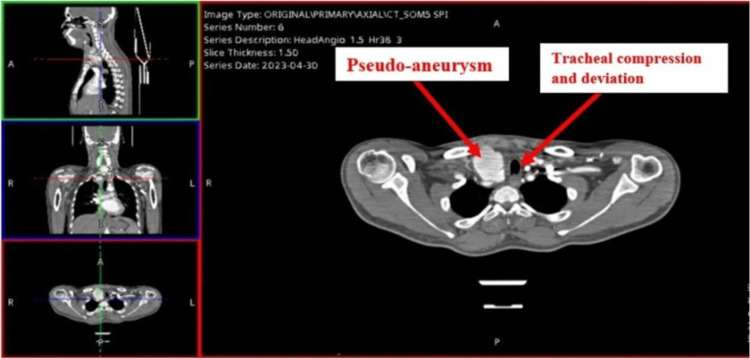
CT-scan showing pseudoaneurysm compressing trachea (axial view on right).
